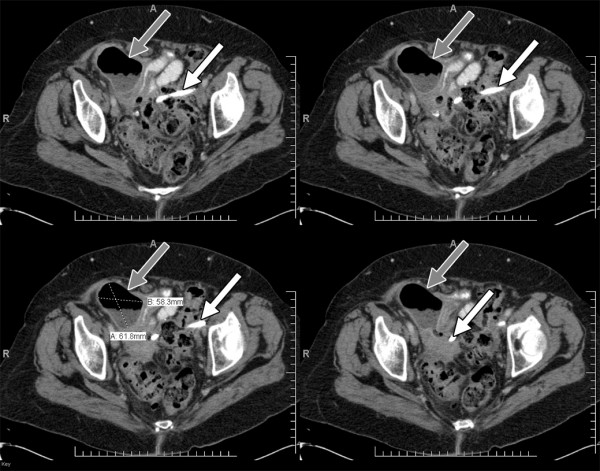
CT Scan with right lower quadrant abscess. Computer tomography images with intravenous and oral contrast demonstrating left lower quadrant abscess and small bowel obstruction. Grey arrows denote the abscess cavity. White arrows denote the endostent.
